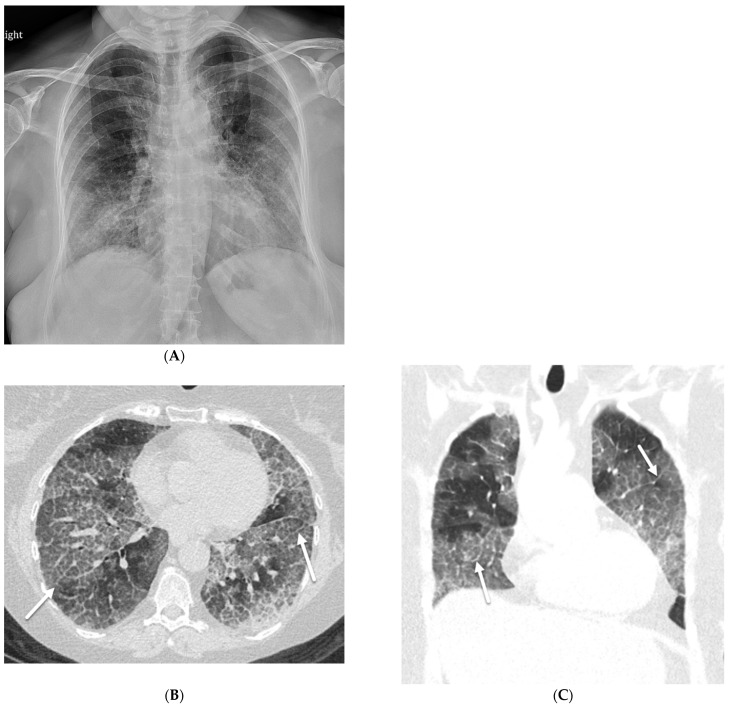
A 54-year-old female with a history of acute myeloid leukemia post allogenic bone marrow transplantation presents with pulmonary graft-versus-host disease. A frontal chest radiograph (A) demonstrates bilateral reticular opacities. Axial (B) and coronal (C) chest CT images reveal basilar predominant ground glass opacities with superimposed smooth interlobular septal thickening (solid white arrow) and intralobular reticulation, giving a crazy paving pattern. The patient was diagnosed with PAP after bronchoscopy.
